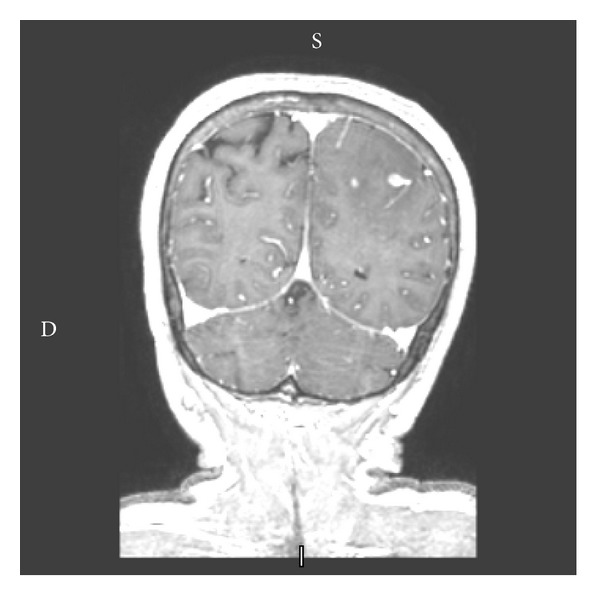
Coronal T1-weighted MR image with gadolinium showing a partially enhancing lesion.
